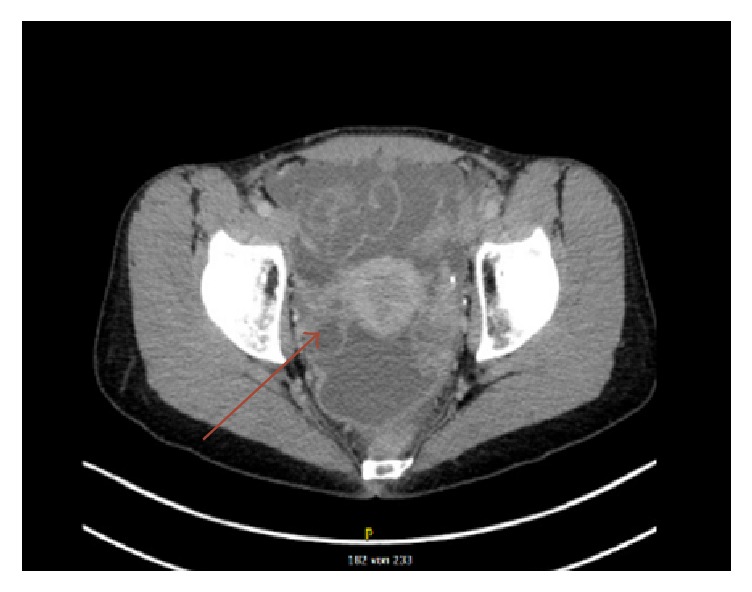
CT scan showing diffuse peritoneal enhancement consistent with peritoneal carcinomatosis, 4-quadrant ascites, and slightly enlarged ovaries with solid and cystic structures. The arrow refers to the right ovary.
